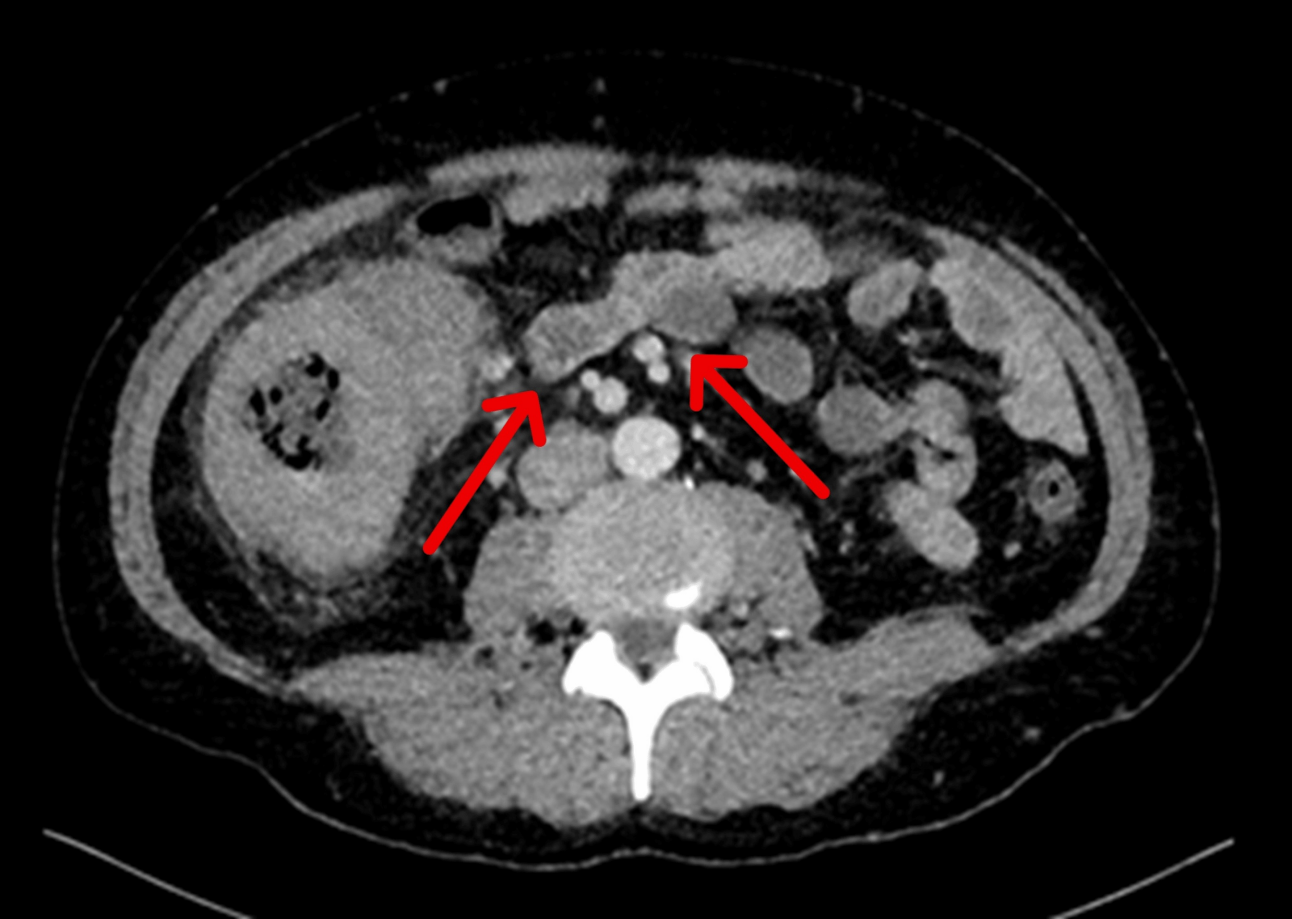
Contrast-enhanced CT scan of the abdomen and pelvis Axial CT image showing diffuse, circumferential wall thickening of the right colon extending to the cecum, ileocecal valve, terminal ileum, and appendix, with pronounced pericolic fat stranding and reactive mesenteric lymphadenopathy. The imaging features were suggestive of a progressive inflammatory or infectious process CT: computed tomography
